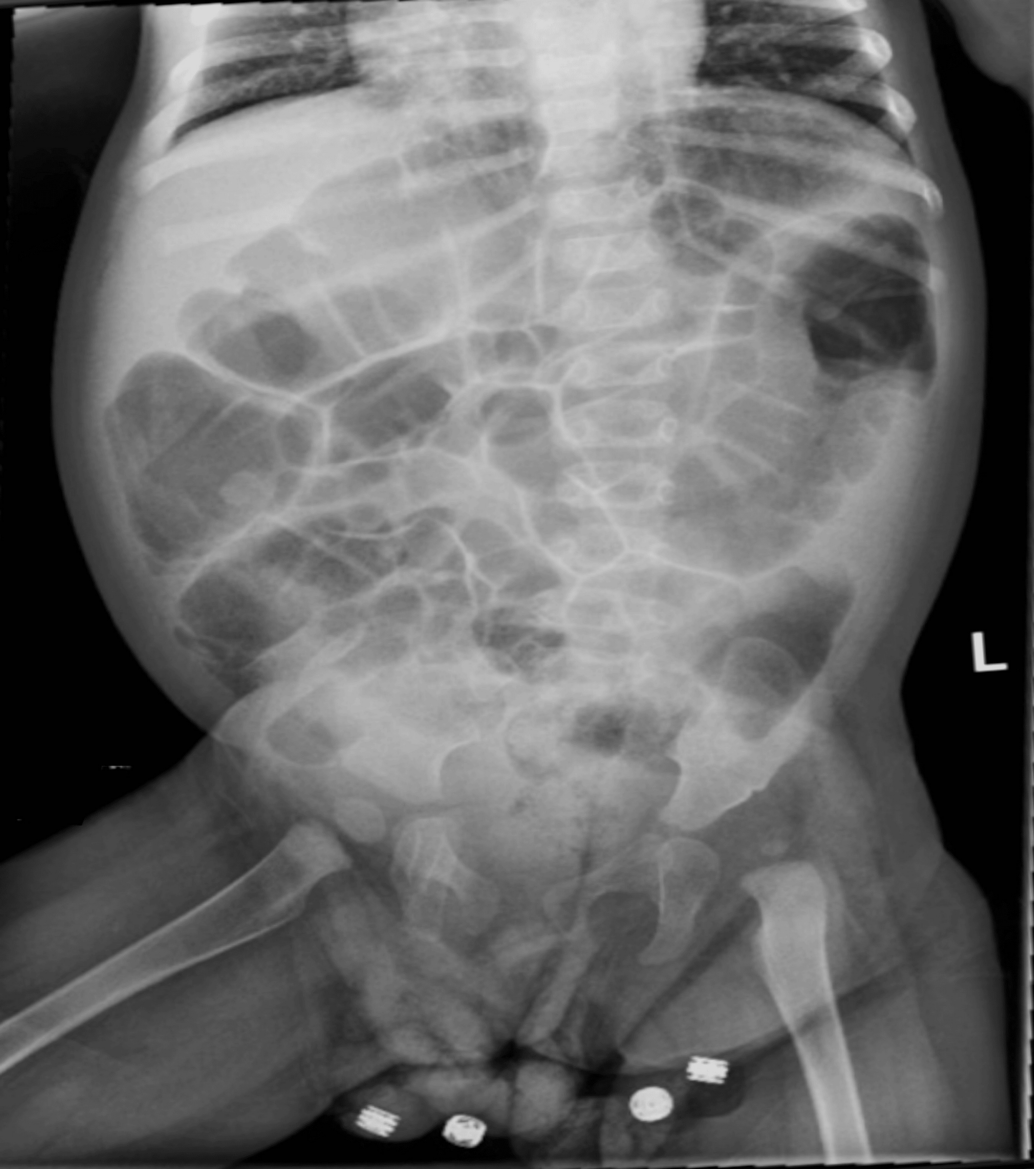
The patient’s abdominal X-ray on her second day of hospitalization revealed no evidence of obstruction, organomegaly, or mass effect. Normal gas pattern throughout. No dilated loops to suggest obstruction. No air-fluid level.
